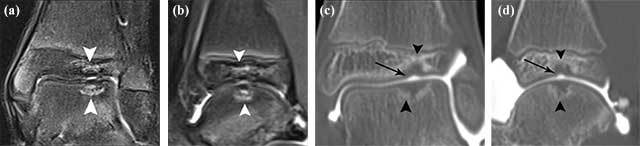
Example of accurate staging of the status of the articular cartilage in paediatric OCL. Coronal (a) and sagittal (b) fat suppressed T2-WI show adjacent kissing areas of bone marrow edema (white arrowheads) at the distal tibia and talar dome. The overlying cartilage is difficult to assess on MRI. Coronal (c) and sagittal (d) reformatted CBCT-A show a focal bony lesion with peripheral sclerosis in the distal tibia and talus. The overlying cartilage is intact at the talus, whereas there is subtle cartilage lesion at the distal tibia (arrow).
